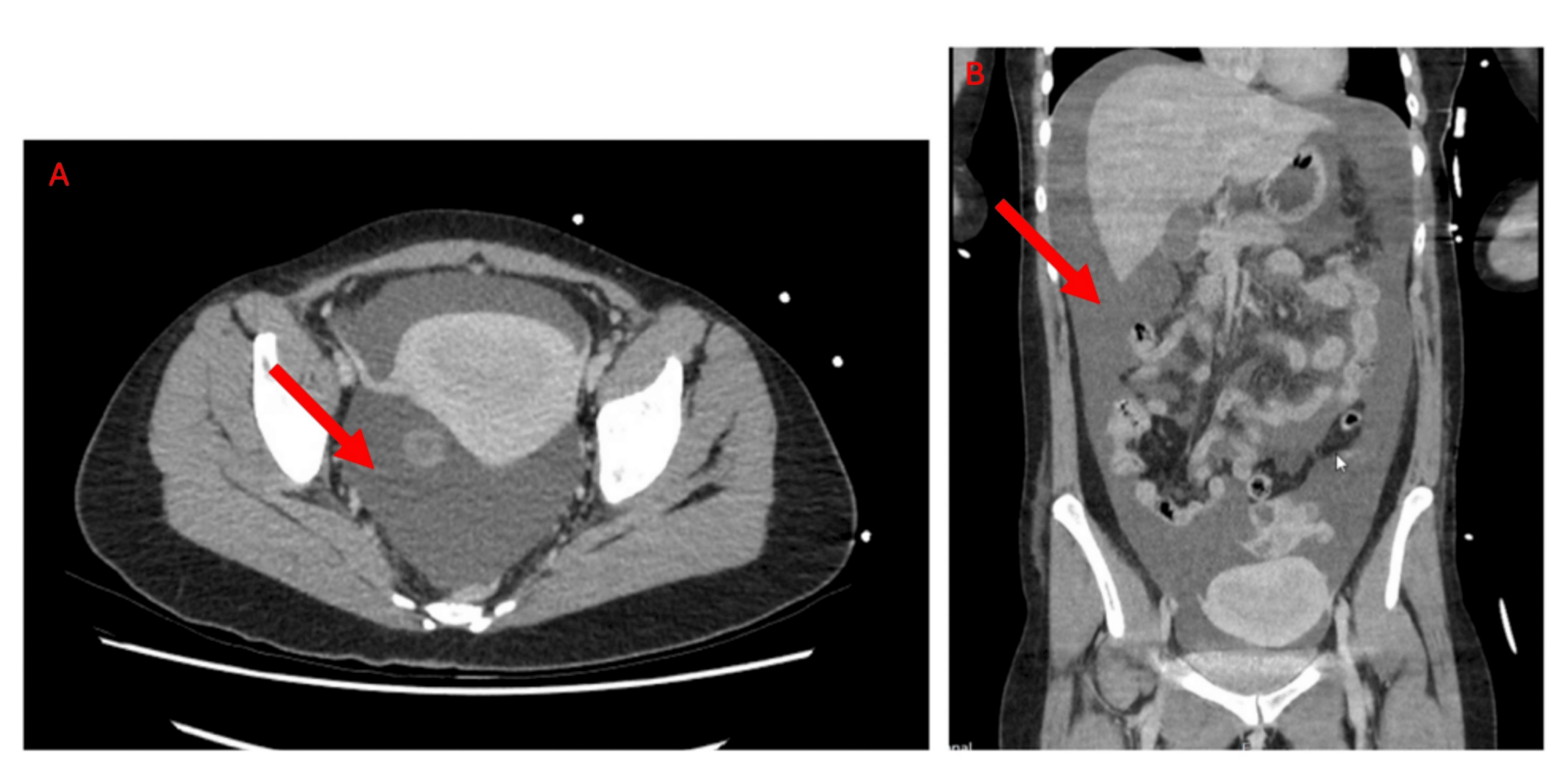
CT of the abdomen and pelvis. (A) Axial contrast-enhanced CT showing free fluid present in the pelvis (red arrow). (B) Coronal CT demonstrating more extensive intra-abdominal free fluid tracking superiorly (red arrow).
